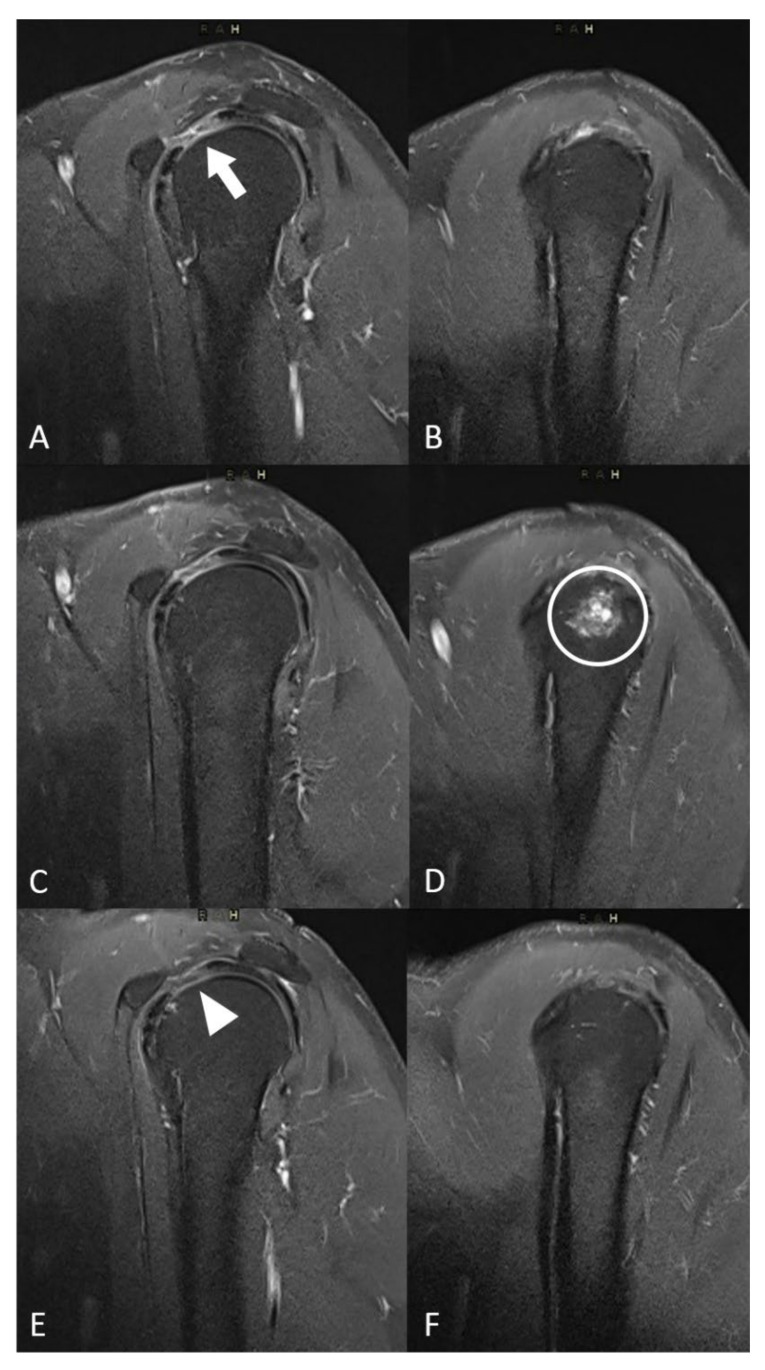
Evolution of MRI inflammatory signal and local complication. (A,B) Sagital T2 fat-sat MR images at baseline showed a hypersignal in the interval of rotator cuff (white arrow). (C,D) Sagital T2 fat-sat MR images one month after embolization showed a liquidian hypersignal in the humeral head (white circle) corresponding to focal oedema. (E,F) Sagital T2 fat-sat MR images 3 months after the procedure showed the decreased inflammatory signal in the interval of rotator cuff (white arrowhead) and the spontaneous disappearance of the humeral head edema.
